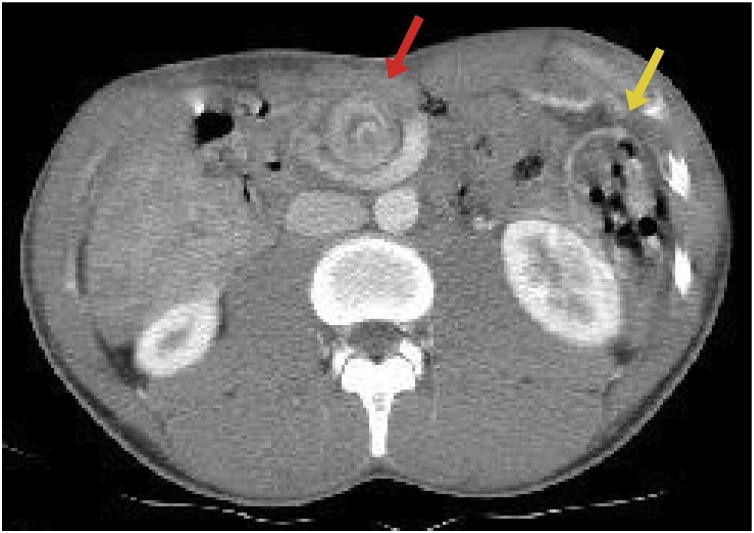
Abdominal CT scan showing aberrant bowel placement (yellow arrow) and mesenteric vessel torsion (red arrow).
